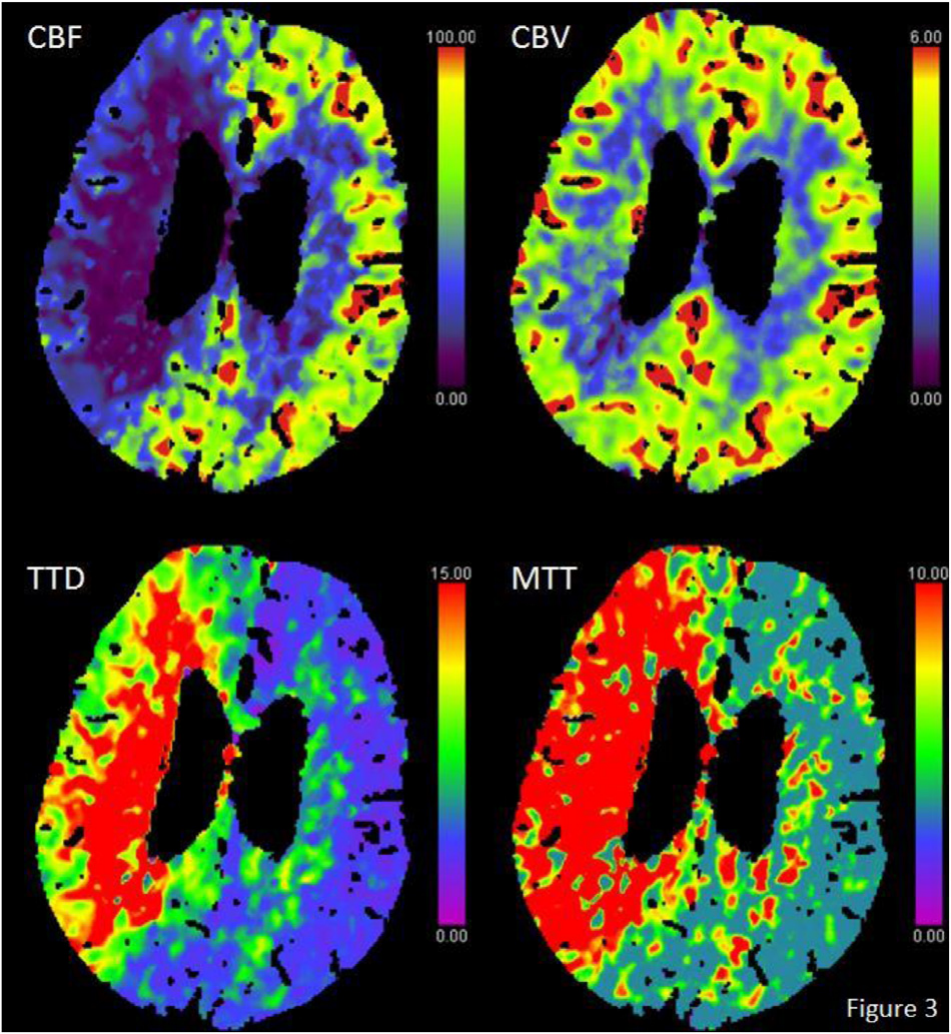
CT Perfusion axial plan view including cerebral blood flow (CBF), cerebral blood volume (CBV), mean transit time (MTT), and time To drain (TTD). CBF shows markedly decreased flow in the right cerebral hemisphere. CBV shows normal blood volume in both cerebral hemispheres. TTD shows markedly increased drain time in the right cerebral hemisphere and slightly reduced drain time in the left cerebral hemisphere. MMT shows markedly increased transit time in the right cerebral hemisphere and slightly reduced transit time in the left cerebral hemisphere.
